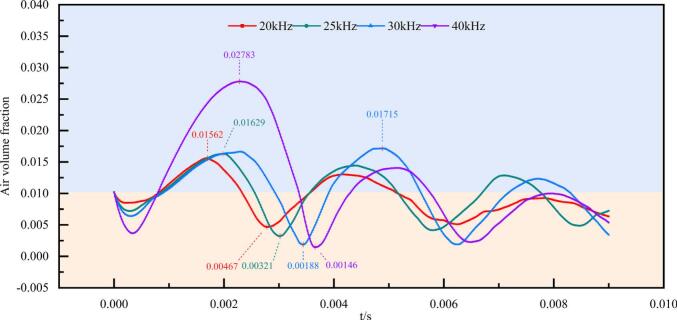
Temporal evolution of air volume fraction under different ultrasonic frequencies (20, 25, 30, and 40 kHz) at a fixed vibration amplitude of 20 μm.
